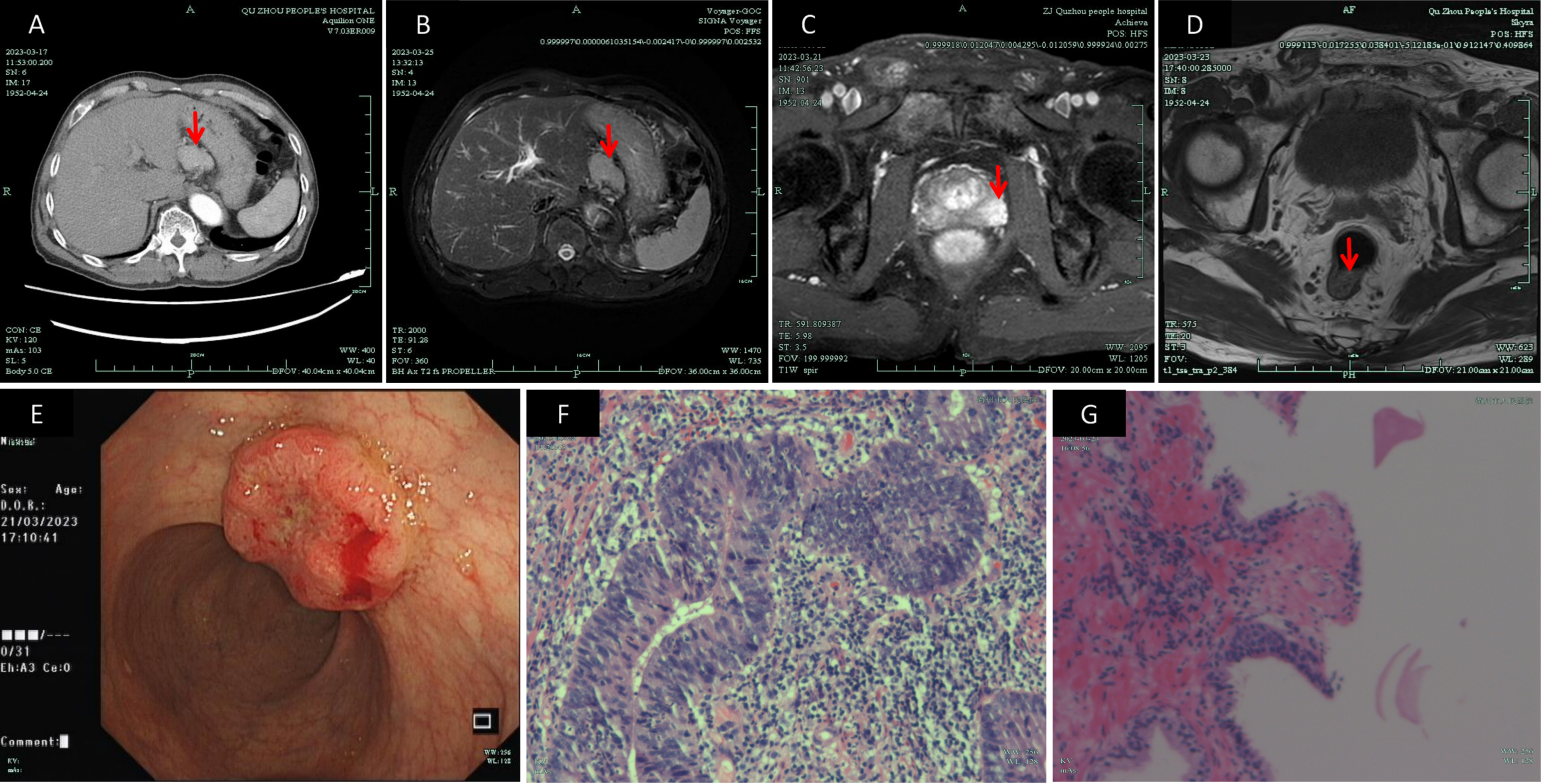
Preoperative imaging studies: (A) CT image of the mass in the hepatogastric space (March 17, 2023); (B) MR image of the hepatogastric lesion (March 25, 2023); (C) MR image depicting an abnormal signal in the left peripheral zone of the prostate and a lesion in the lower rectum (March 21, 2023); (D) MR image used for rectal cancer staging (March 23, 2023); (E) Colonoscopic image of the rectal mass (March 21, 2023); (F) Pathological image from colonoscopic biopsy confirming rectal adenocarcinoma (March 24, 2023)(×400); (G) Pathological image from transperineal prostate biopsy confirming prostate adenocarcinoma (March 24, 2023)(×100). CT, Computed Tomography; MR, Magnetic Resonance.
